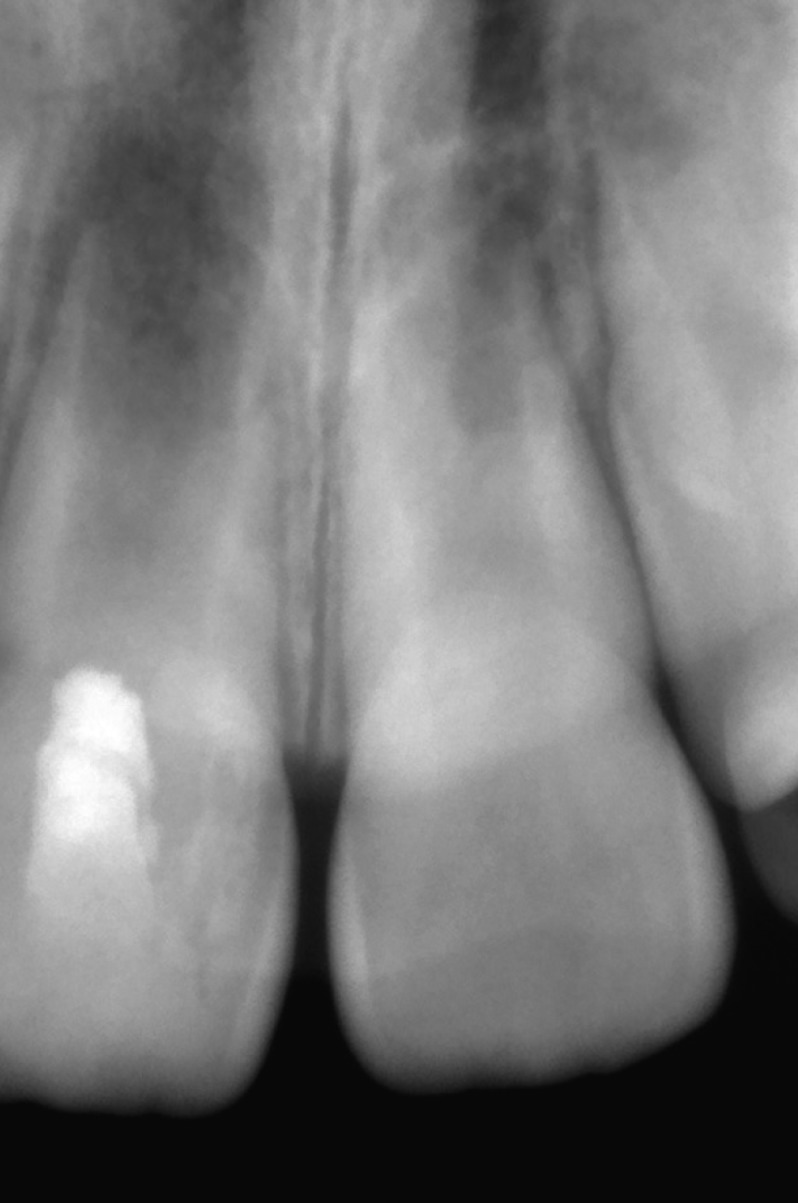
Diagnostic intraoral x-ray of the central incisor with failed revascularization treatment. As visible, mesial pulp horn was not included to access cavity design.
